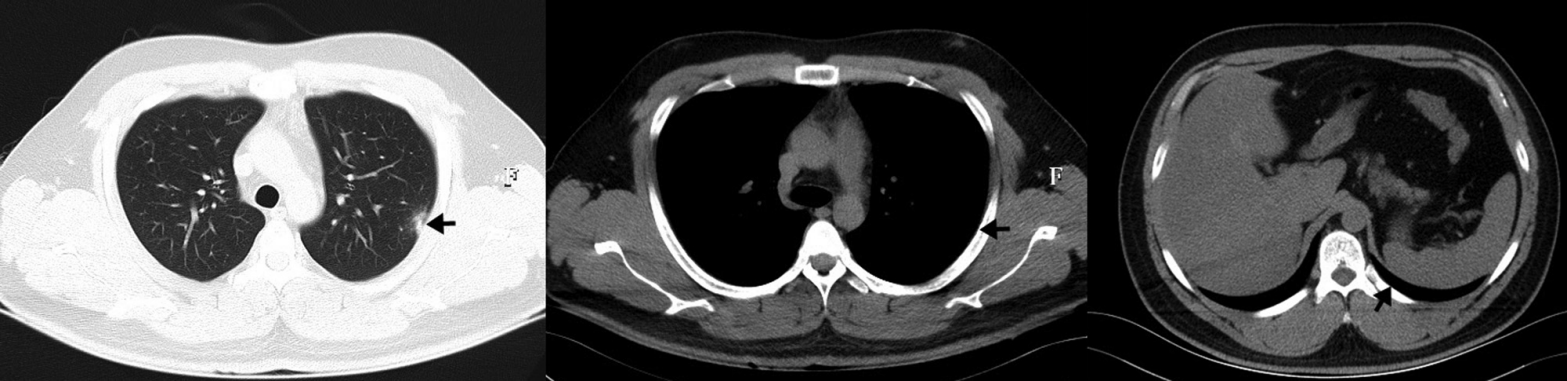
Chest CT after 6 weeks of treatment, showing the cavity disappeared, leaving only a little patchy infiltration. The pleural thickening was improved.
